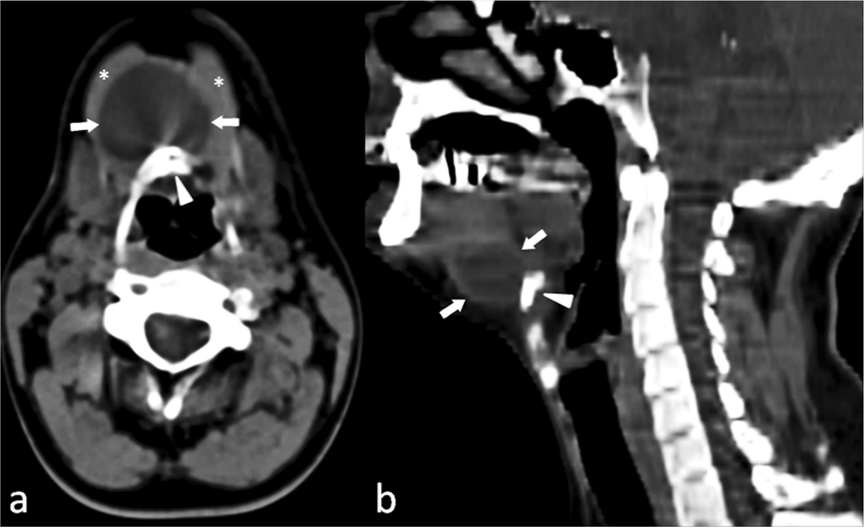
Axial (a) and sagittal (b) unenhanced computed tomography scans show the presence of a hypodense round mass (3 × 4 cm) (arrows) with a fluid density value (mean density = 20 HU) located medially and in front of the hyoid bone (arrowhead).
